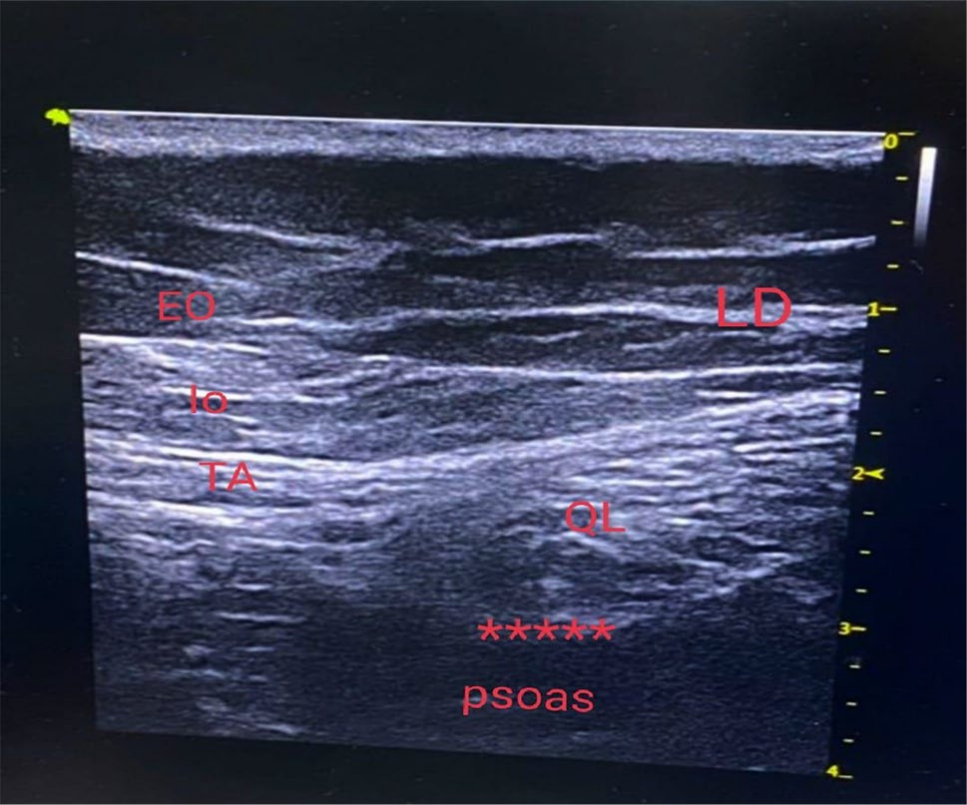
Ultrasound guided quadratus lumborum block. _*_: site of injection. QL: quadratus lumborum; EO: externak oblique; IO: internal oblique; TA: transversus abdominis; LD: latismus dorsi
